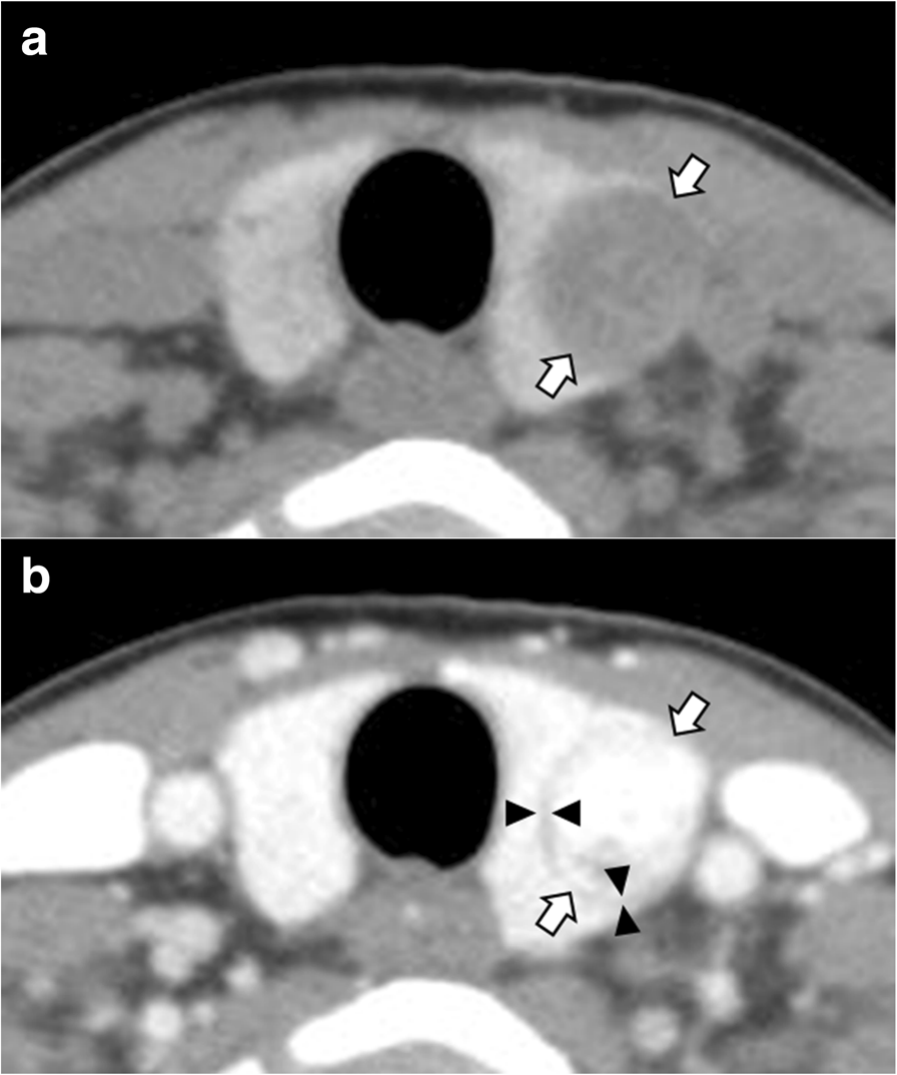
A 51-year-old woman with follicular adenoma in the left thyroid lobe (largest diameter, 1.9 cm). In non-enhanced (a) and contrast-enhanced (b) axial CT images, follicular adenoma (arrows) in the left thyroid lobe shows homogeneous low attenuation, mildly expansile configuration, taller-than-wide shape, inhomogeneous and increased enhancement, and presence of the CT halo sign (arrowheads)
